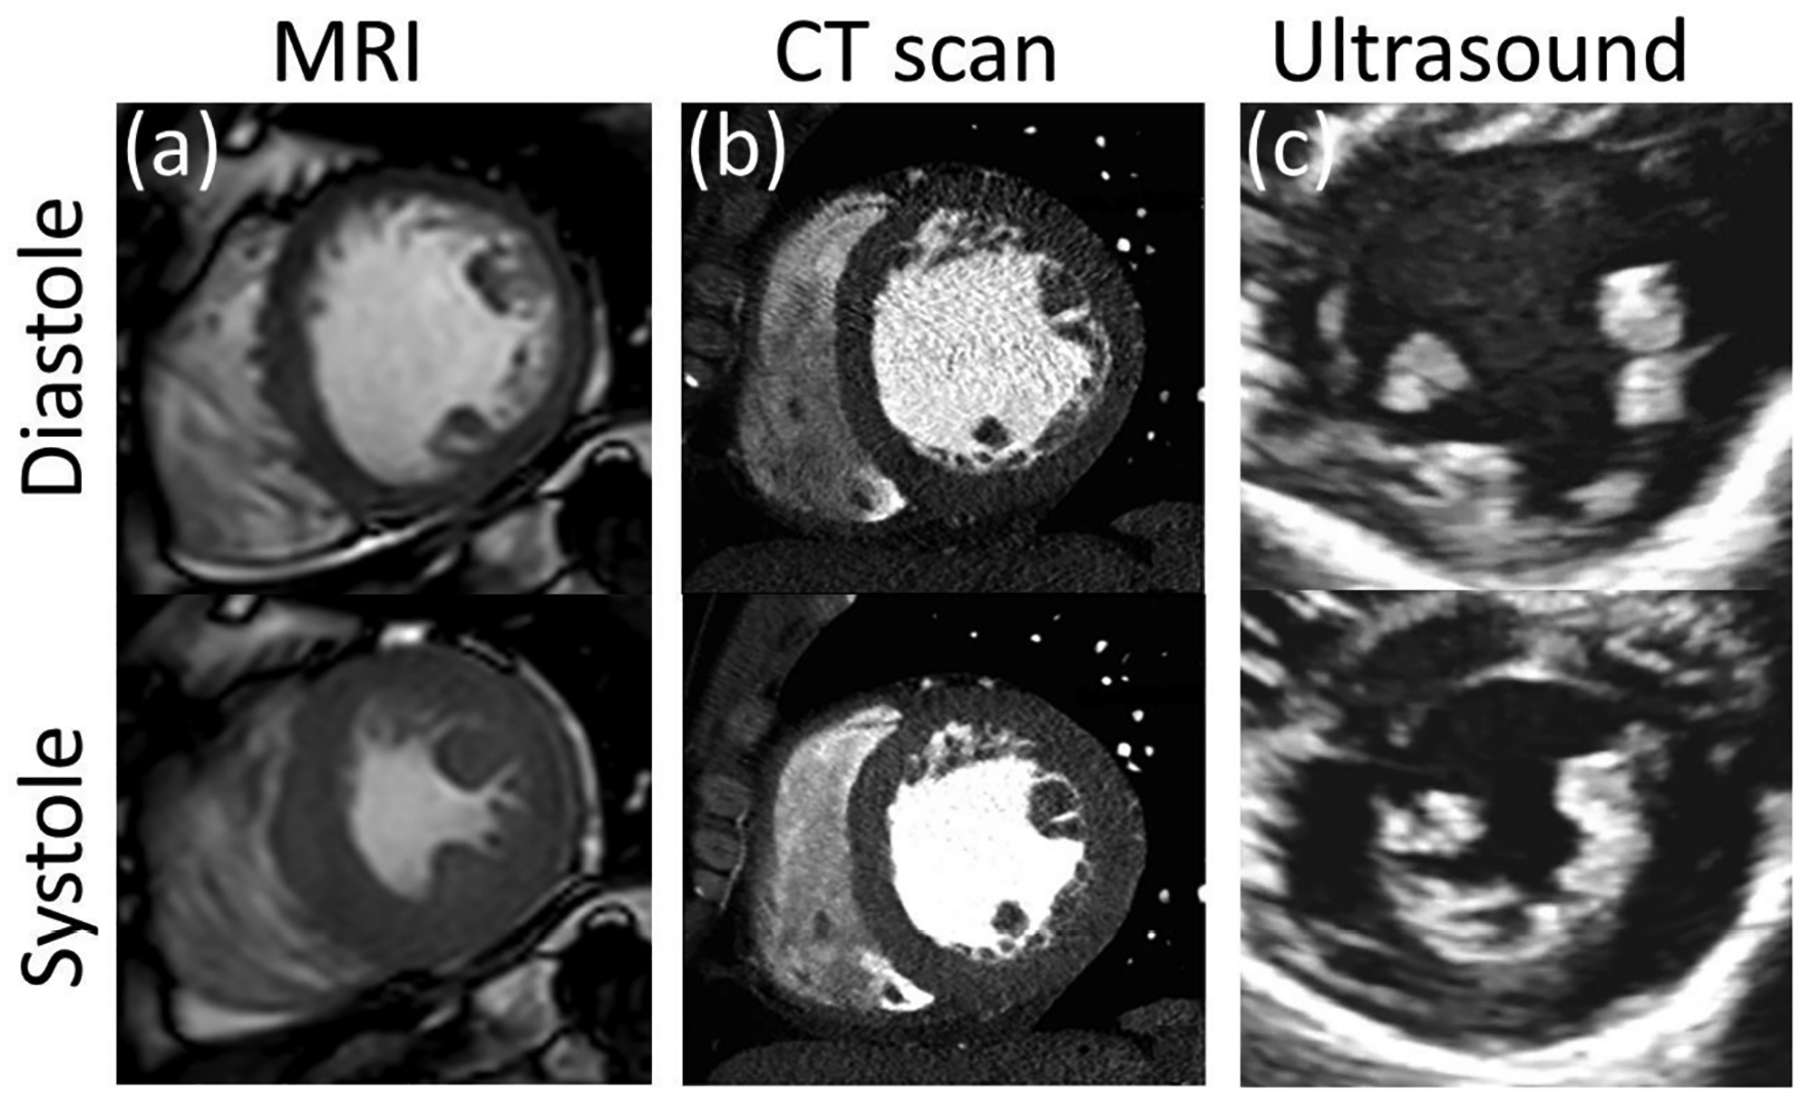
Resolution comparison of left ventricular myocardium at diastole and systole with clinical grade (a) MRI, (b) CT (Reprinted/adapted with permission from Ref. [158], 2019, Korean Society of Echocardiography, open access), and (c) 2D ultrasound.
